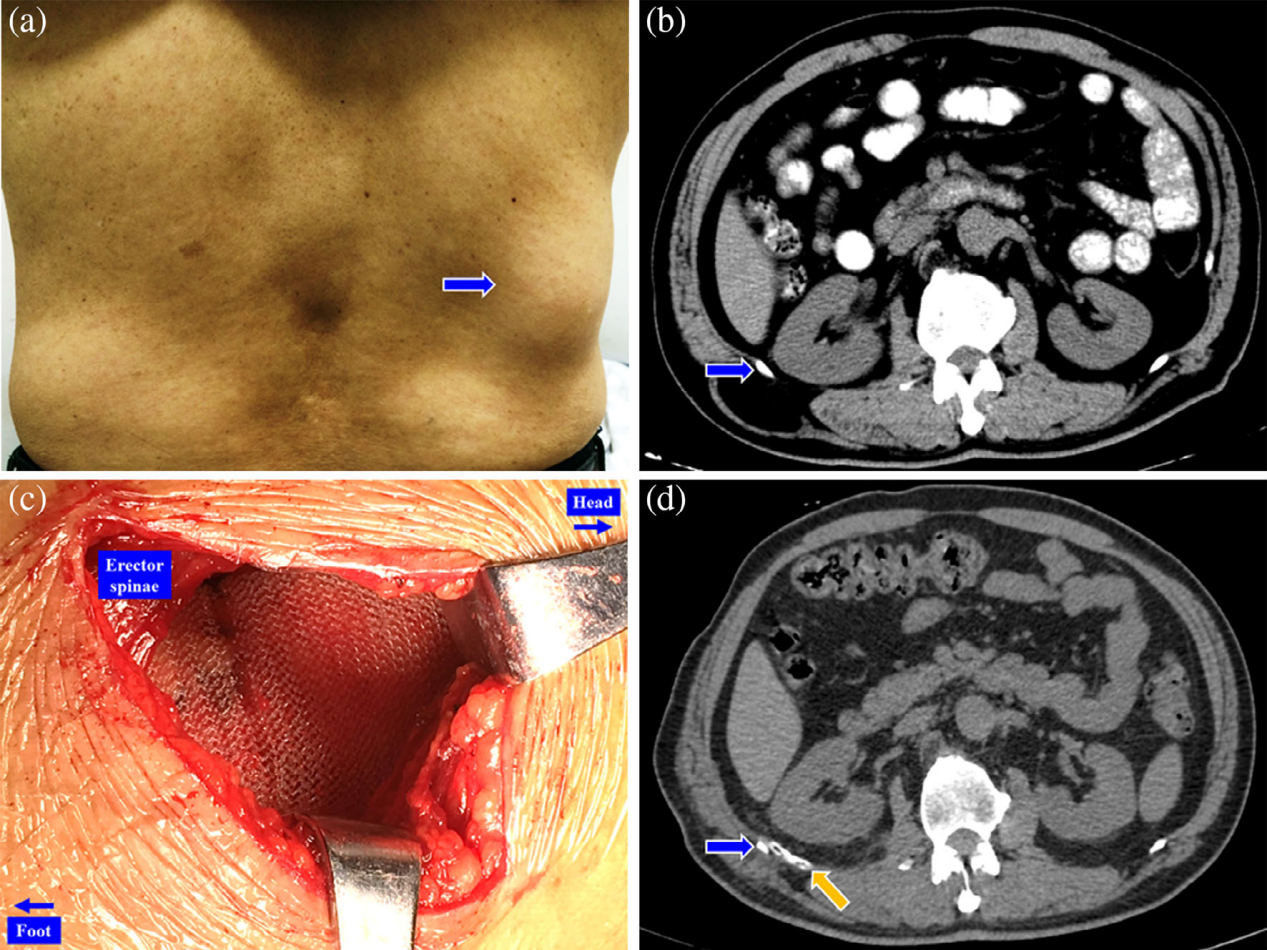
Peri‐operative pictures of a right primary superior lumbar hernia. (a) A physical examination of a right primary superior lumbar hernia (blue arrow). (b) Preoperative computed tomography (CT) scan of a right primary superior lumbar hernia, the blue arrow indicates the 12th rib. (c) Placement of the Kugel patch in the retroperitoneal space. (d) Post‐operative CT scan of a right primary superior lumbar hernia, the blue arrow indicates the 12th rib, while the orange arrow indicates the drainage tube.
